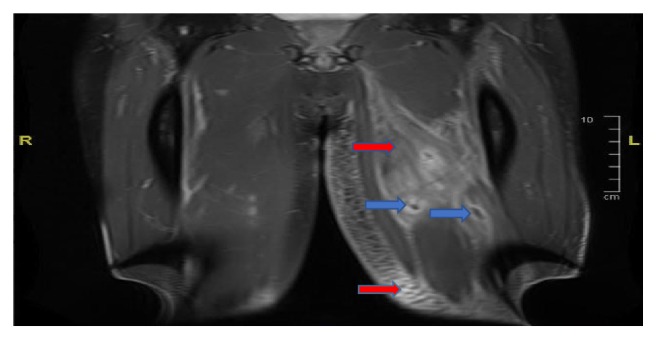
MRI postcoronal T1 of bilateral thighs. Red arrows show extensive subcutaneous edema with enhancement throughout the subcutaneous adipose space of the left thigh as well as extensive infiltration of muscles in multiple compartments with blue arrow showing abscess.
